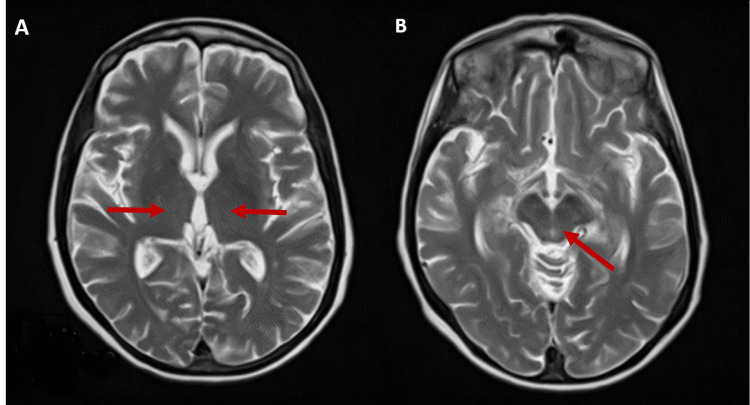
Brain MRI Diffuse cortico-subcortical atrophy, predominantly frontoparietal, with no noticeable signal changes in the brain parenchyma, particularly in the thalamus (A), hypothalamus, mammillary bodies or midbrain (B) (T2-weighted image).
